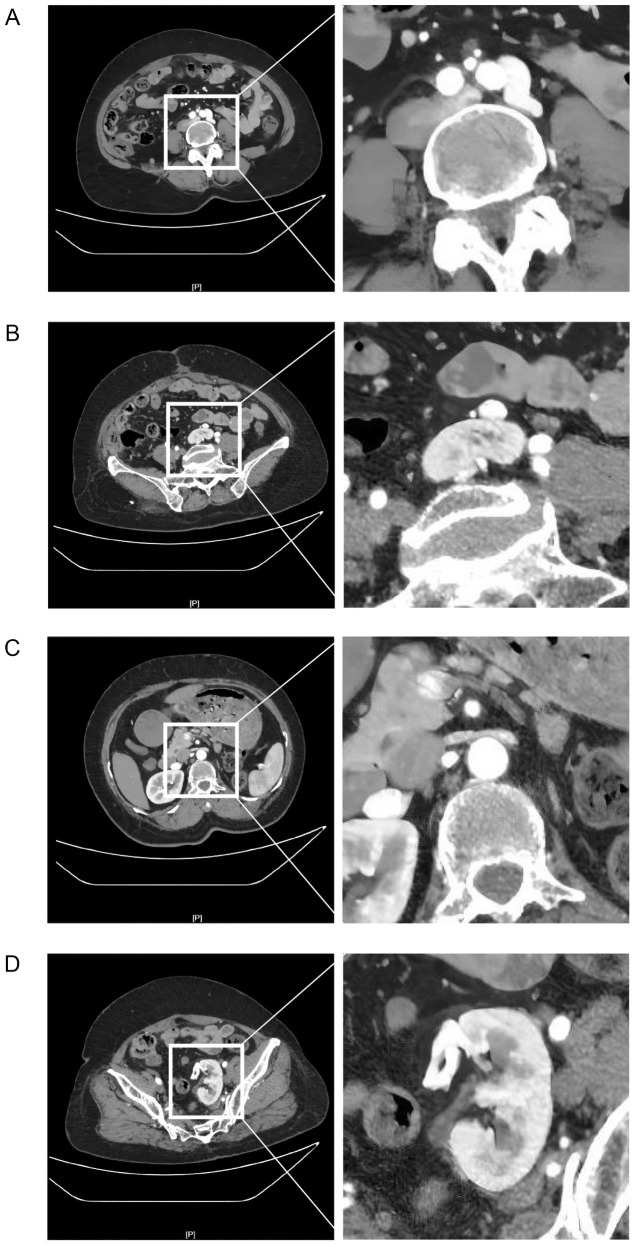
Variations in vascular anatomy of the left pelvic ectopic kidney on contrast-enhanced CT. (A) The left renal artery aberrantly originates from the left common iliac artery. (B) The left renal artery enters the renal hilum via the upper pole of the kidney. (C) The left renal vein drains into the inferior vena cava at a normal anatomical position. (D) The left renal vein divides into multiple branches at the renal hilum for venous drainage.
